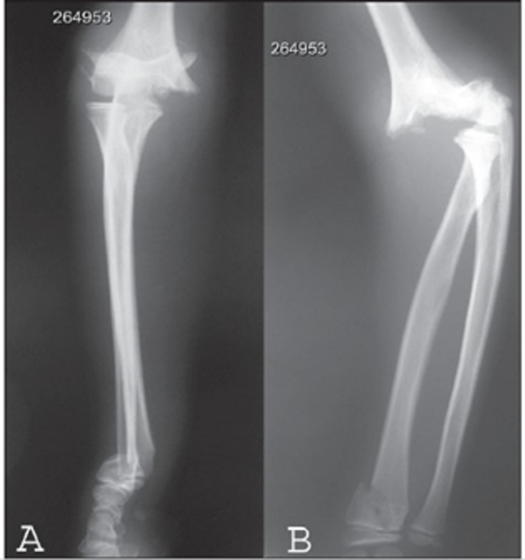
(A) X-ray of the elbow and forearm shows supracondylar with distal radius fracture. (B) Lateral view shows gross displacement of supracondylar fracture
